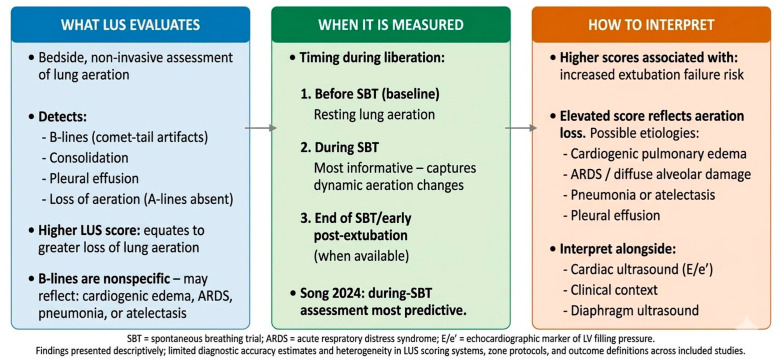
Descriptive summary of lung ultrasound (LUS) findings during spontaneous breathing trials. Higher LUS scores reflect aeration loss from multiple possible etiologies including ARDS, pneumonia, atelectasis, and cardiogenic edema. Data from [3,6]. SBT = spontaneous breathing trial; ARDS = acute respiratory distress syndrome.
